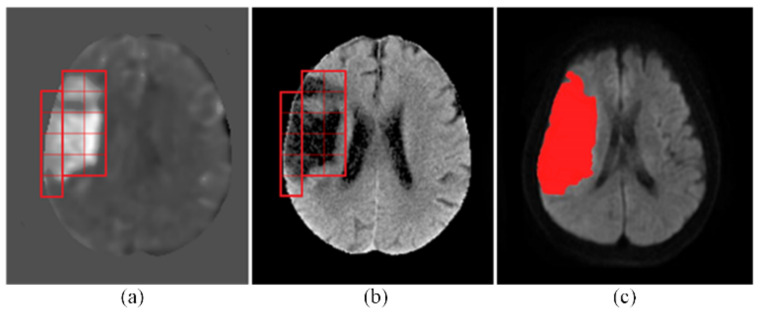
An exemplary result of infarct detection by the proposed CNN. (a) The detected infarcted patches were marked with red squares on the t-score map. (b) They were also marked on the intensity-transformed brain parenchyma image, i.e., the CT image after Step P5. (c) The red area on the MRI represents the detected infarcted region delineated by the experienced neurologist.
